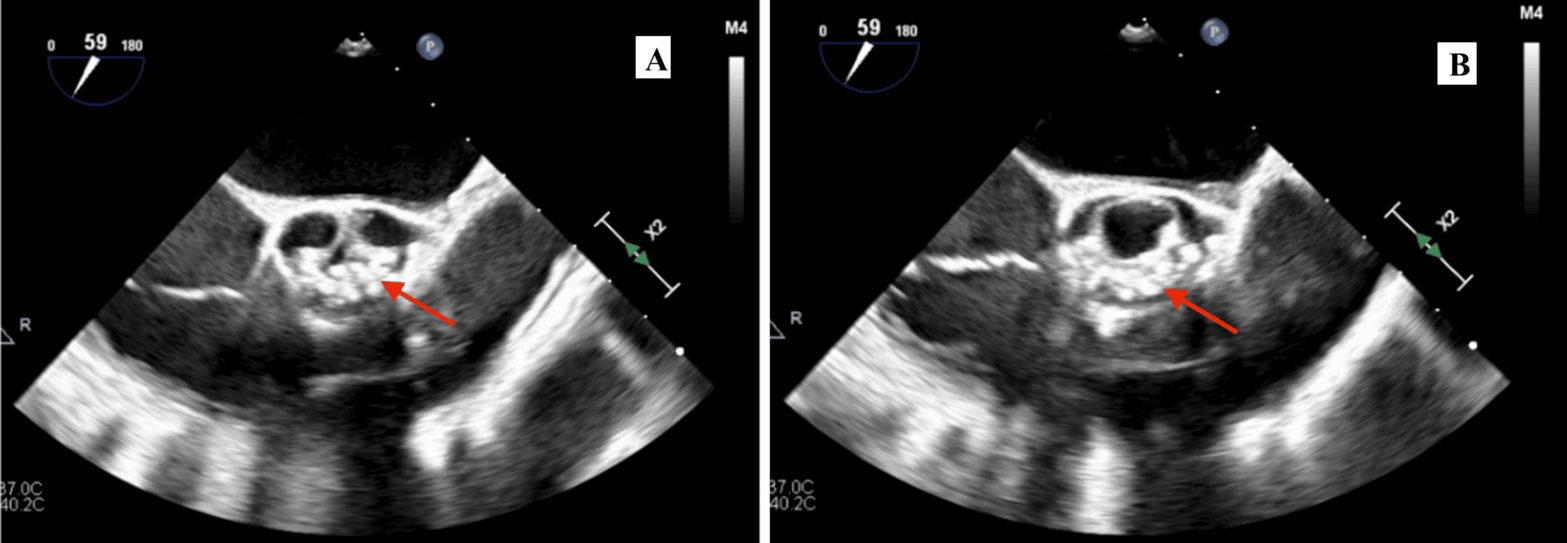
Transesophageal echocardiogram in the midesophageal short-axis view showing a severely calcified unicuspid aortic valve. The red arrows indicate the fused commissures.
